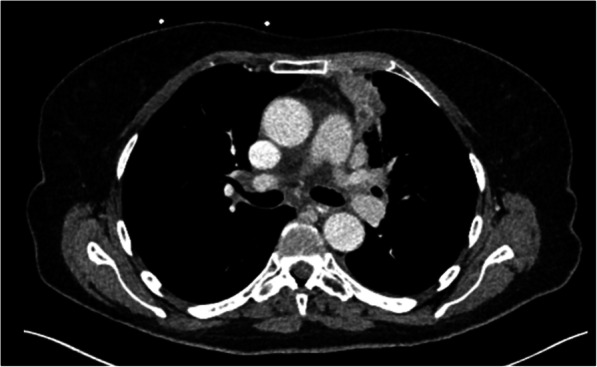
CT appearance of left upper lobe primary tumour
